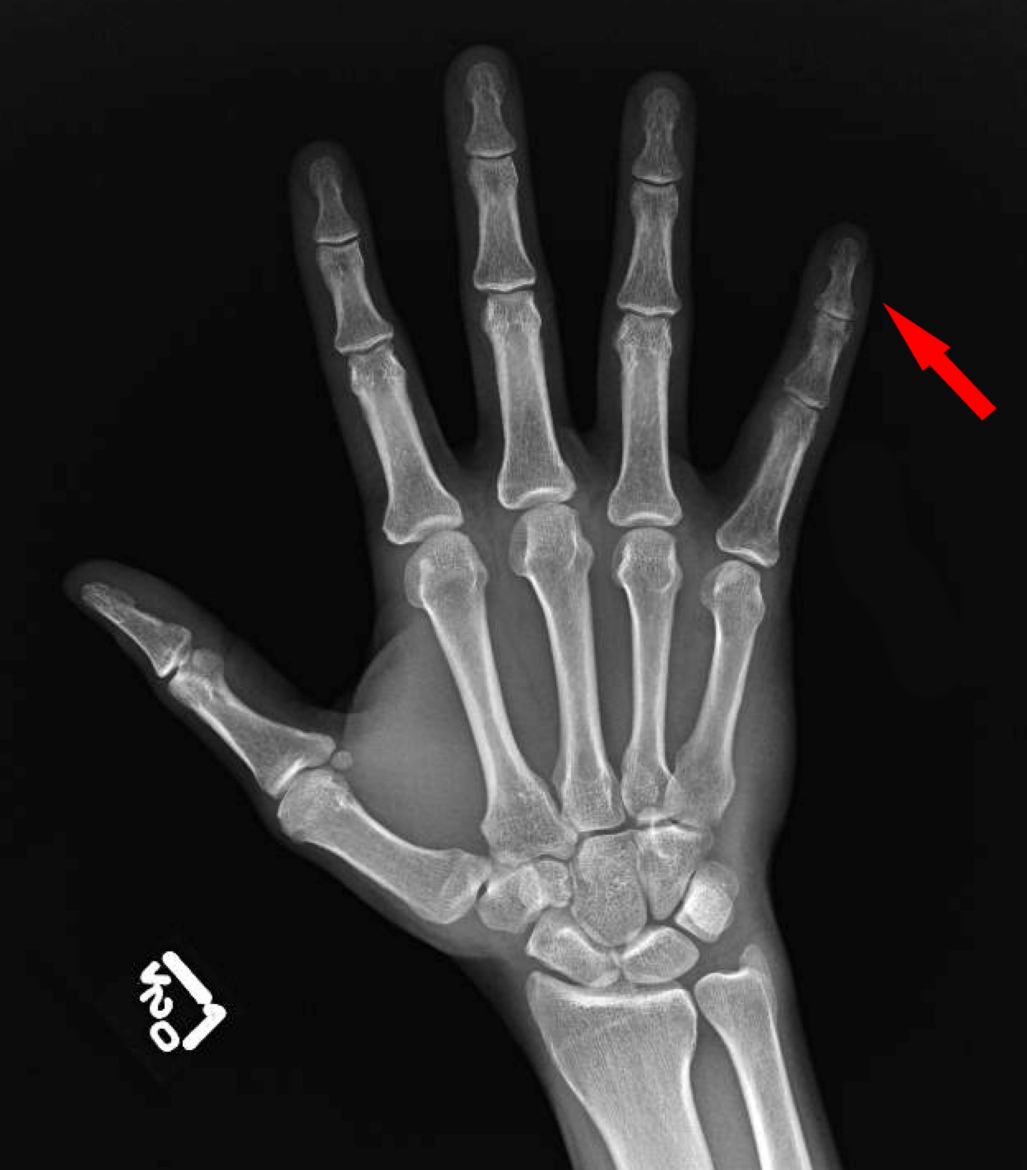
X-ray dorsal view of the left hand three months following crush injury.
